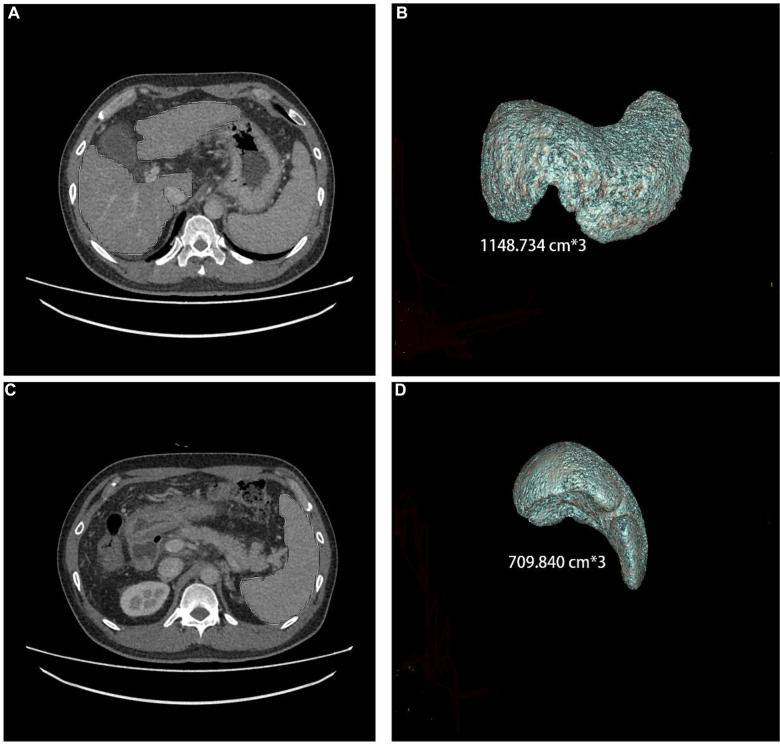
Liver and spleen volume, as measured by CT, in a 44-year-old male who has had hepatitis B cirrhosis for 20 years.
